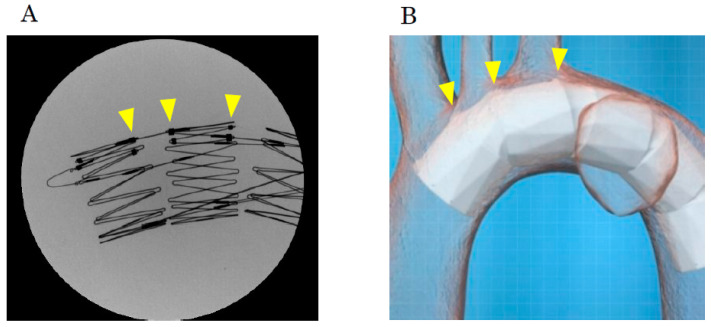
(A) Under radiographic fluoroscopy, the position of the fenestrations can be confirmed using multiple markers where stent struts are connected on the greater curvature side (arrows). (B) When the stent markers are aligned with the arch vessels according to the placement plan, the fenestrations naturally align with the arch vessels (arrows).
